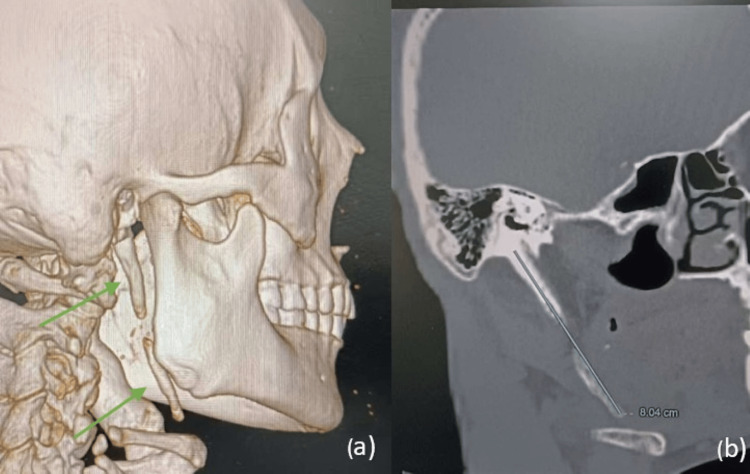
(a) 3D-reconstructed view of the right styloid process; (b) Sagittal cone-beam computed tomography (CBCT) slice showing measurement of the right styloid–stylohyoid complex (8.04 cm)
